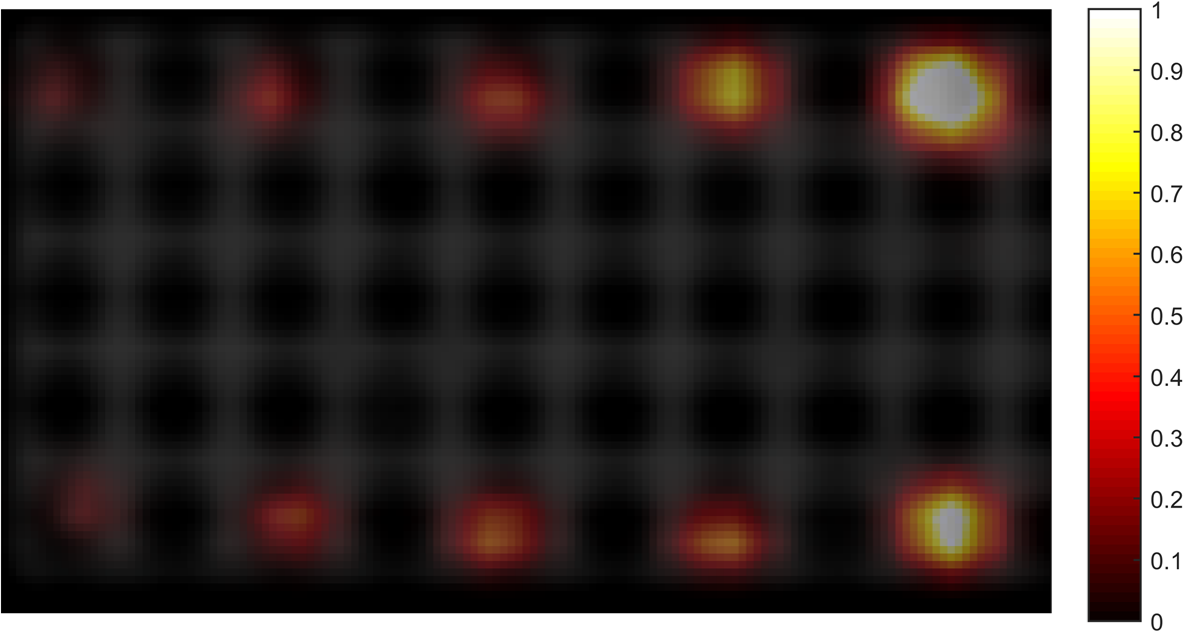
PET/CT image of 10 different Eppendorf tubes, all containing 1 × 10^5^ LNCaP cells in 1 ml media and incubated at different concentrations of Ga68-PSMA-11. First row of tubes from left to right, 193, 338, 544, 780, and 996 nM radioligand concentration, respectively (replicated in the second row)
